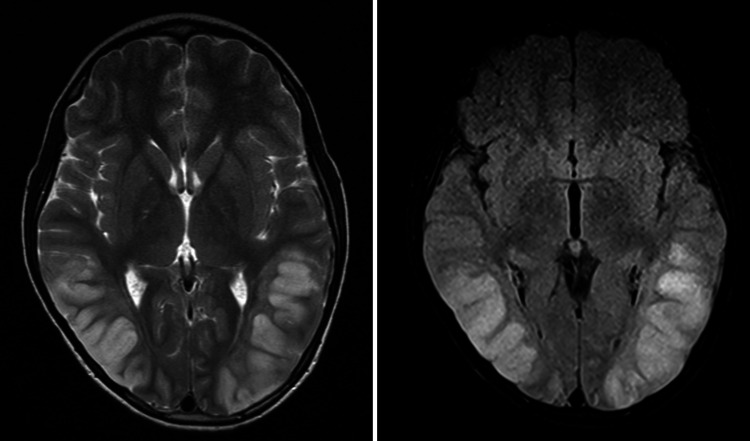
Brain magnetic resonance imaging on hospital admission. Subcortical and cortical hyperintensity in the occipital and parietal lobes in T2 and FLAIR sequences. FLAIR, fluid-attenuated inversion recovery.
